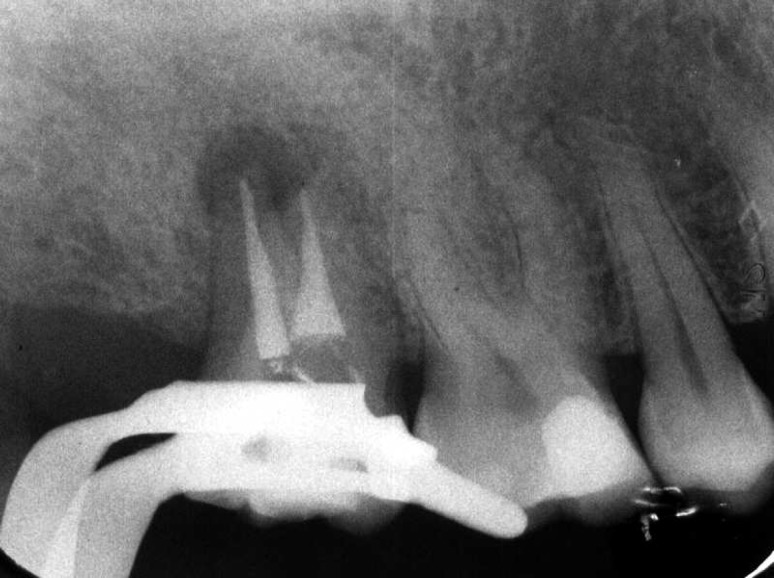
Postobturation radiograph
